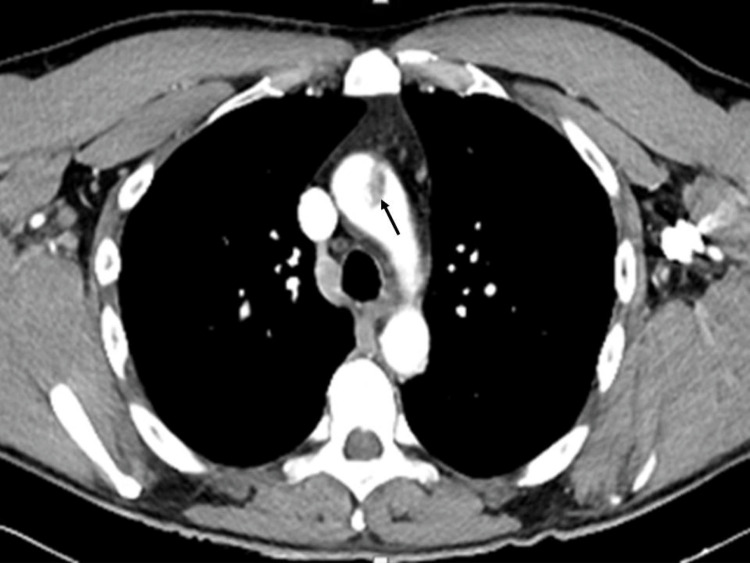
CTA showing an endoluminal repletion defect in the ascending aorta (axial view) CTA: computed tomography angiography
